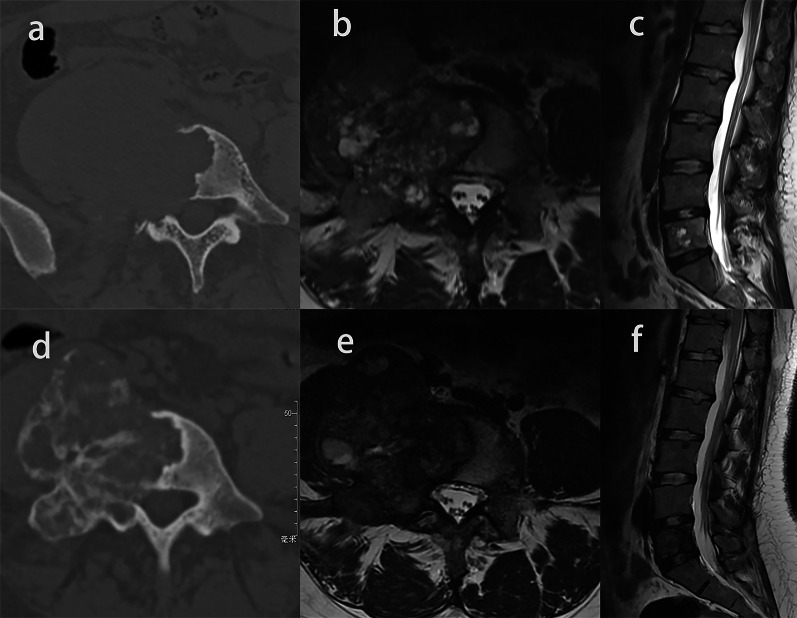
Image of a giant cell tumor of L5 in a 23-year-old woman. a, d Axial CT scans before and after denosumab treatment. Incomplete peripheral rim and bone formation inside the mass over 50% of the transverse session are observed (grade 2b). b, e Axial and sagittal MR images before and after denosumab treatment. The diameter of the cyst is smaller. c, f Sagittal MR images before and after denosumab treatment, the signal intensity (SI) ratio between the solid part of the GCTB and the normal spinal cord on T2-weighted MR images was decreased
